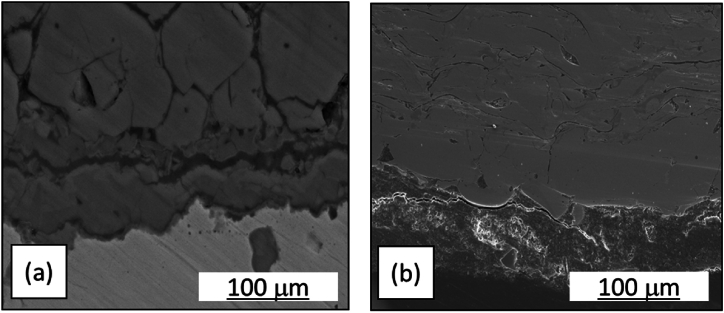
Micro photo of the cross-sections of a) WT, b) CTA.
